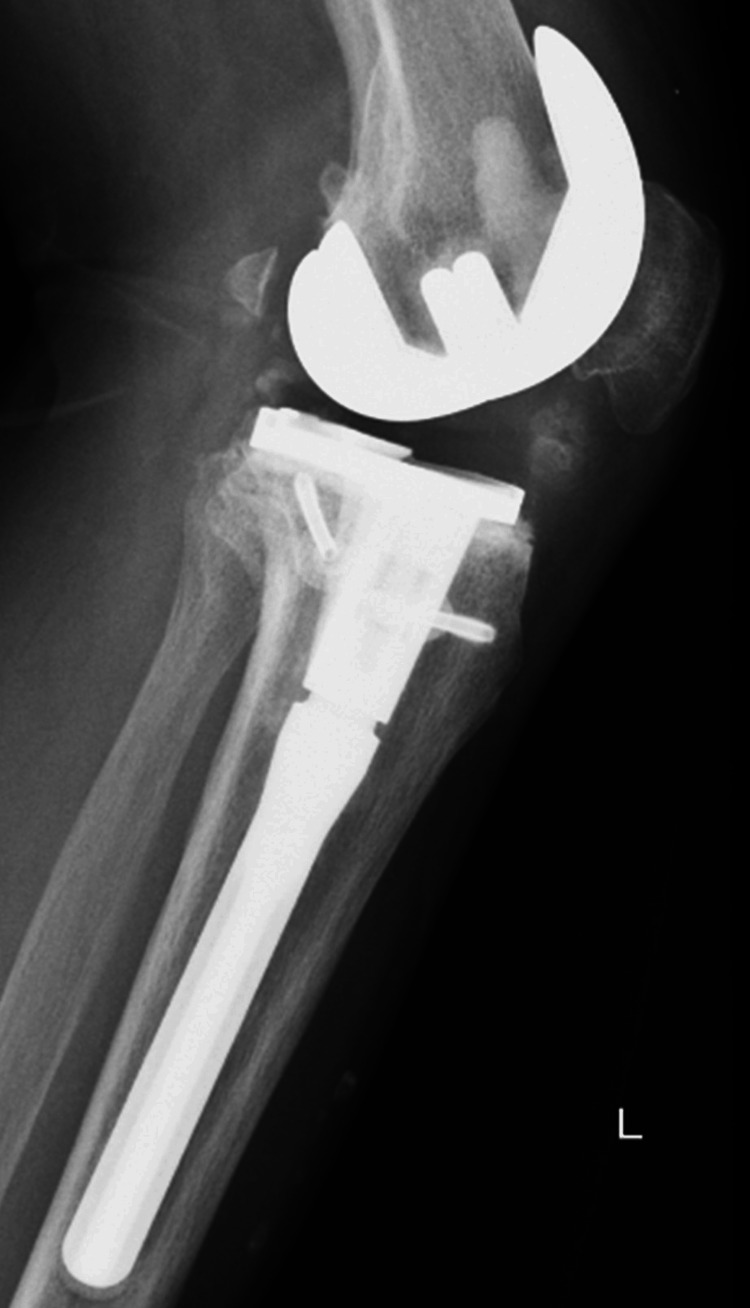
Two years postoperative radiography of TKA (lateral view) TKA: total knee arthroplasty.
